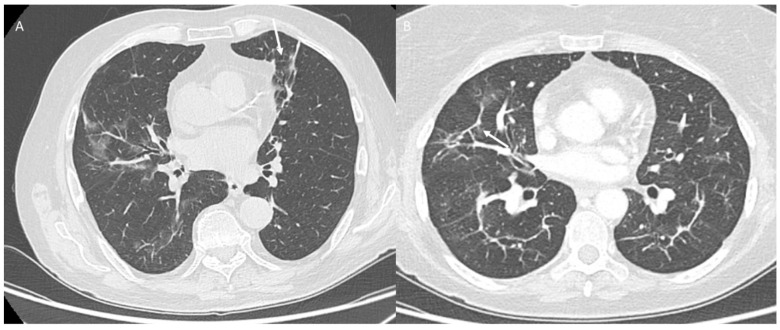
COVID-19 patient at late stage. CT (axial plane: (A,B)) shows fibrotic-like changes (arrow).
